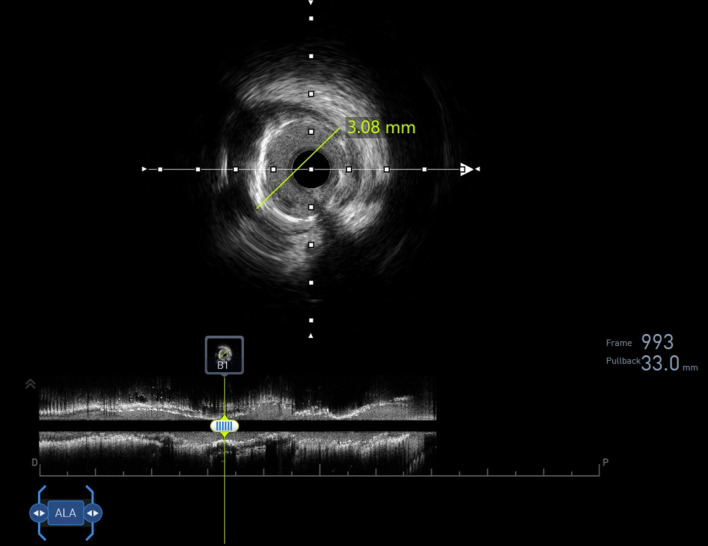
Intravascular ultrasound confirming 99% in-stent thrombosis in the proximal left anterior descending (LAD) stent, with minimal lumen area of 1.2 mm^2^.
